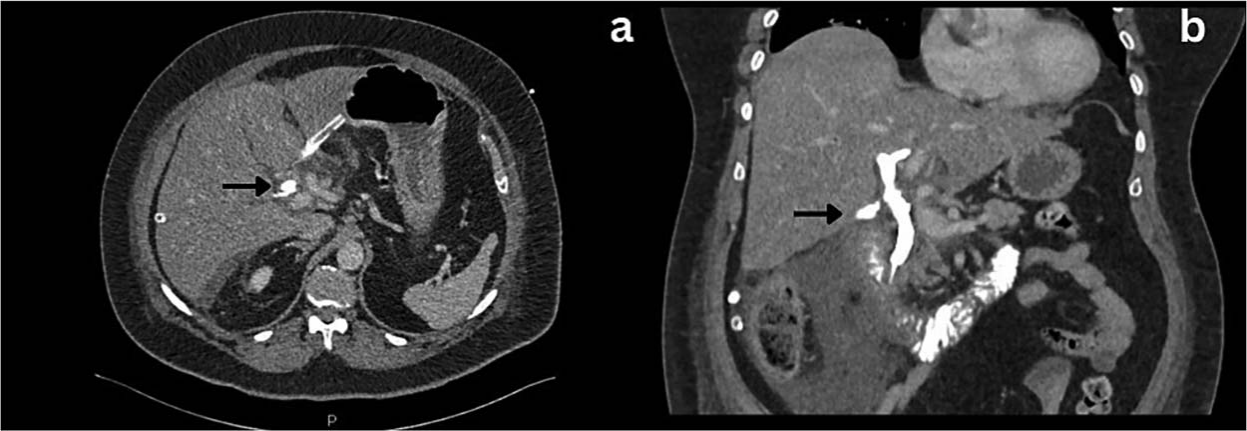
Axial and coronal section CT imaging revealing contrast leak from the bile duct; a) Contrast leak from the posterior aspect of CBD (arrow). b) Contrast extravasation into the subhepatic drain (arrow).
